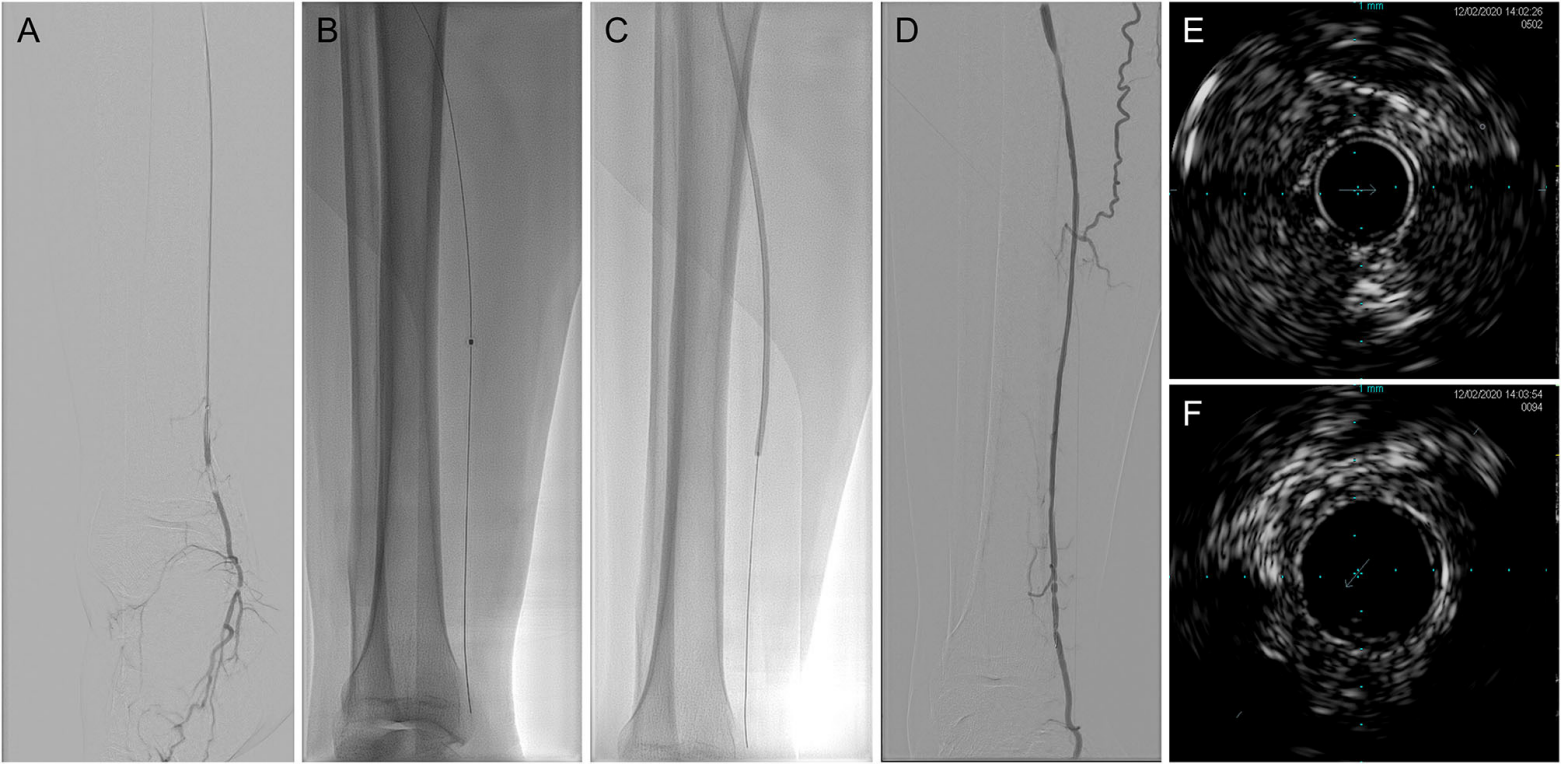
Representative digital subtraction angiography (DSA) and intravenous ultrasound (IVUS) imaging of laser-assisted angioplasty intervention. (A) Baseline angiography; (B) excimer laser angioplasty; (C) balloon angioplasty (BA); (D) after intervention angiography; (E) baseline IVUS imaging; and (F) after intervention IVUS imaging. DSA, digital subtraction angiography; IVUS, intravenous ultrasound.
